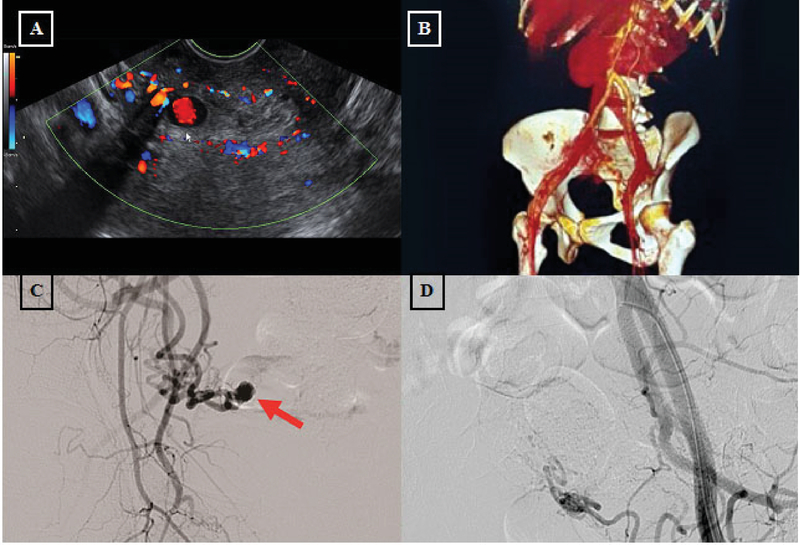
(A) Doppler study showed hematoma connected with right uterine artery; (B) Hyper vascular pattern originated from right uterine artery; (C) Aneurism originated from right uterine artery pre-TAE; and (D) Post micro-coil insertion.
